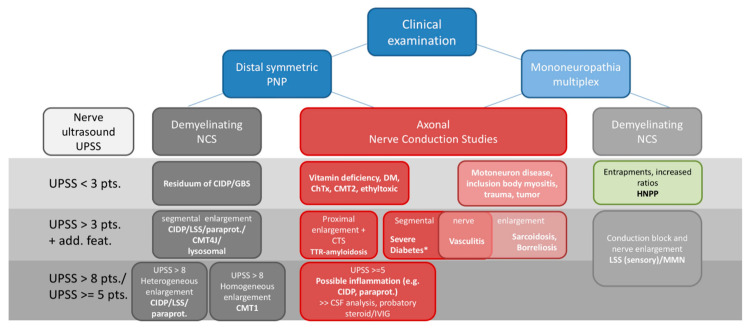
Proposed diagnostic algorithm and possible, most common differential diagnosis for clinical routine including clinical examination, nerve conduction studies and nerve ultrasound. * an additional inflammatory component might play a role in nerve enlargement. Abbreviations: ChTx = chemotherapy, CIDP = chronic inflammatory demyelinating polyneuropathy, CMT = Charcot Marie Tooth, CSF = cerebrospinal fluid analysis, CTS = carpal tunnel syndrome, DM = diabetes mellitus, HNPP = hereditary neuropathy with pressure palsies, GBS = Guillain-Barre-Syndrome, IVIG = intravenous immunoglobulin, LSS = Lewis-Sumner Syndrome, MMN = multifocal motor neuropathy, NCS = nerve conduction studies, paraprot. = paraproteinemic polyneuropathies, PNP = polyneuropathies, TTR = hereditary transthyretin related amyloidosis, UPSS = ultrasound pattern sum score.
